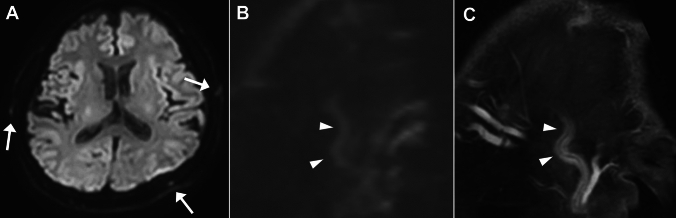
Diffusion-weighted image (DWI) in giant cell arteritis. A 57-year-old man with headache. (A) DWI shows hyperintensity of the bilateral temporal and left occipital arteries (arrows). (B) Oblique sagittal reconstruction of DWI shows hyperintensity of the temporal artery as seen in wall enhancement on (C) fat-suppressed contrast enhanced T1WI (arrowheads in B and C)
